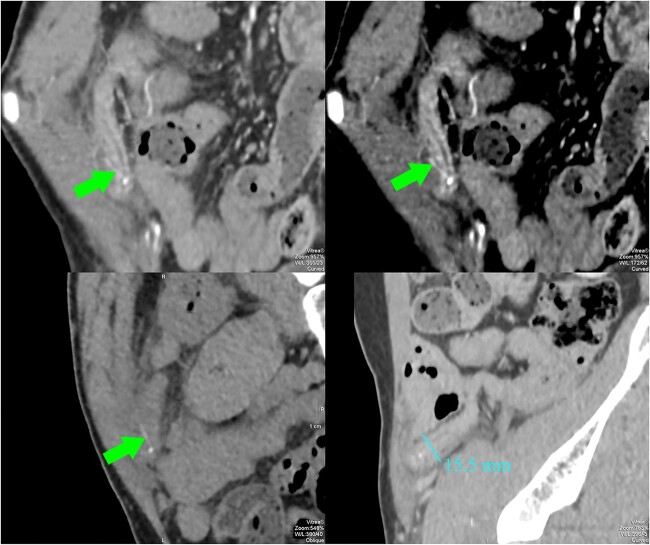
CT images showed a dilated appendix (maximum diameter 15.5 mm) with thickened and hyperenhancing wall. A small amount of free fluid and fat stranding was seen in the right iliac fossa. There was a linear hyperdensity measuring 13 mm (arrows) perforating and extending slightly beyond the appendix. No abscess or free gas collection was detected.
